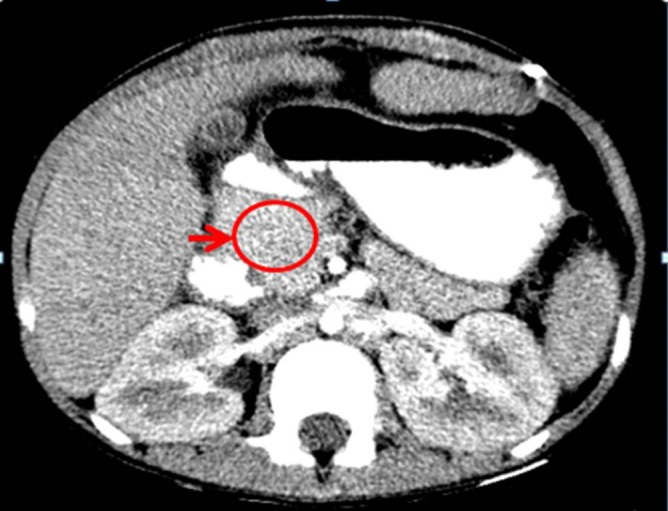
Figure 1:CT scan showing mass lesion in the vicinity of distal CBD and pancreatic head.
